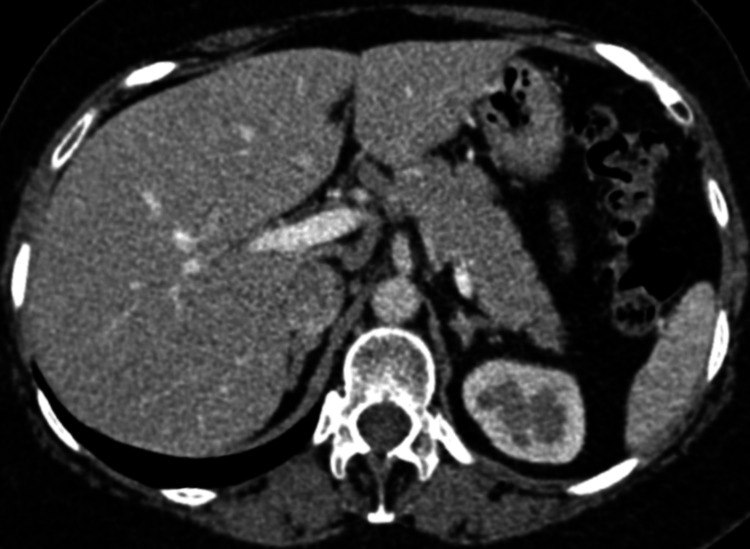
Resolution of chest wall cystic lesion with rib erosion at five-month follow-up. Axial contrast-enhanced CT image, in the soft tissue window, obtained at five-month follow-up, demonstrates complete resolution of the cystic lesion. Minimal, persistent erosion of the underlying ninth rib can be seen anterolaterally. CT: computed tomography
